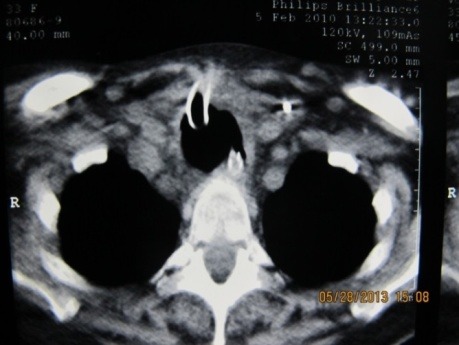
CT scan of a giant trachea-esophageal fistula. Large communication and total destruction of the cartilaginous structure of the trachea
